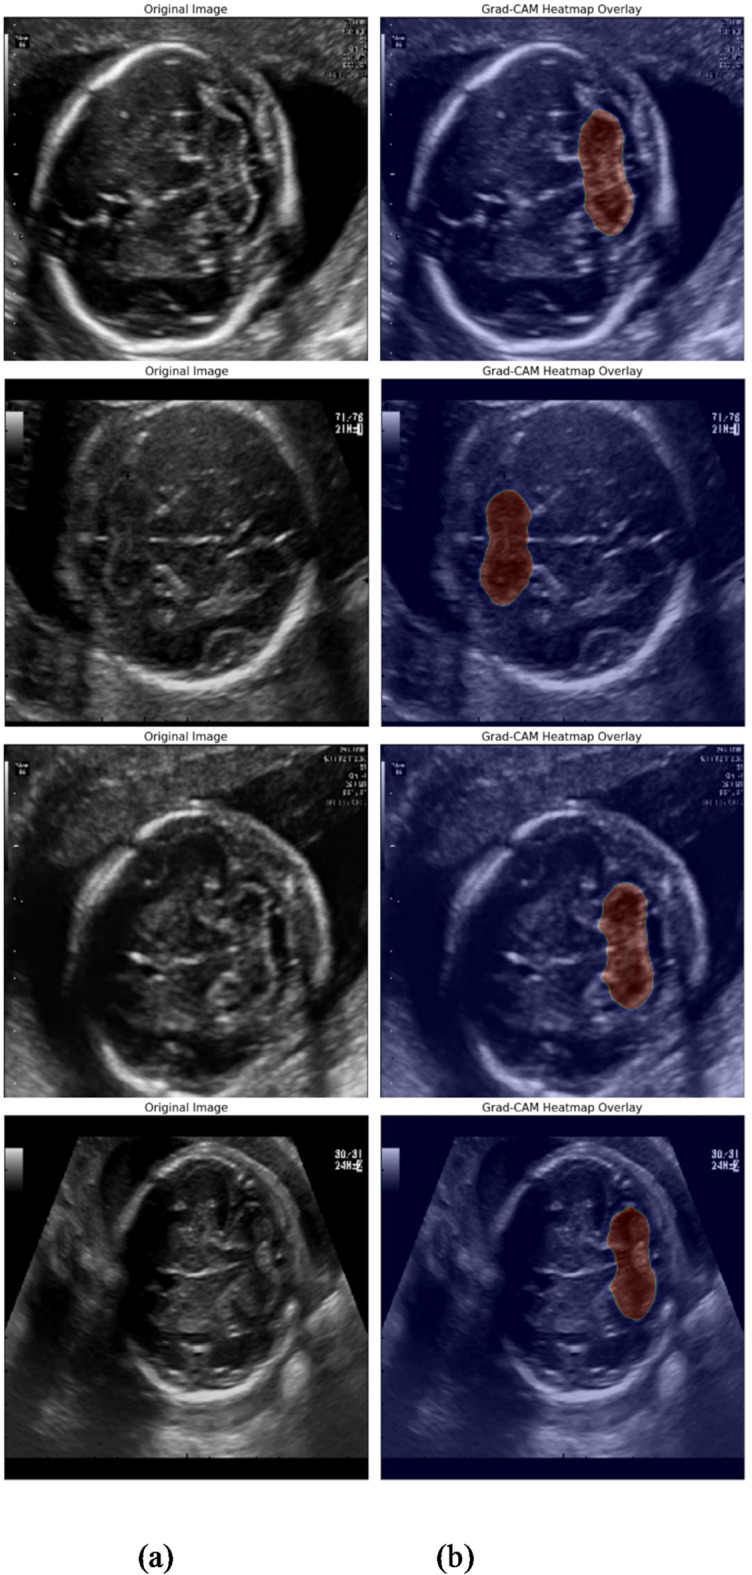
Visual representation of the SS_CASE_UNet model’s performance using heatmaps. (a) Original ultrasound image, and (b) Grad-CAM heatmap.
